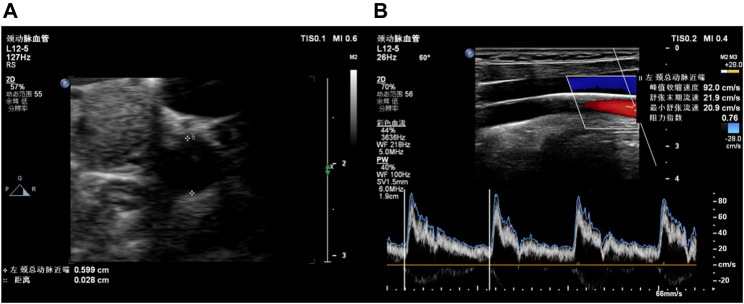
Examples of carotid ultrasound. (A) B-mode ultrasound for IMT; (B) Doppler ultrasound for RI.
